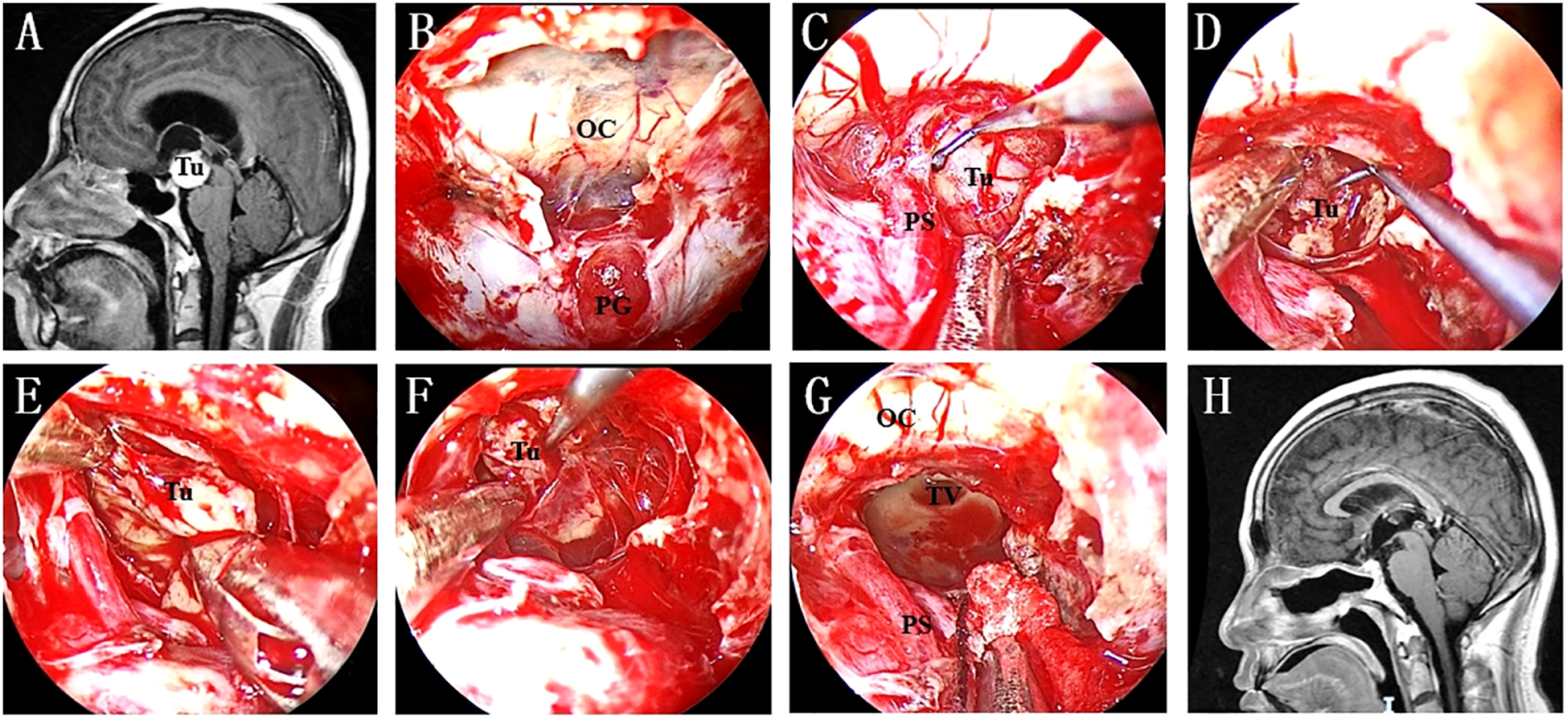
(A) Preoperative MR image showing a lesion in the retroinfundibular area. (B) A “Y”-shaped dural incision was made to expose the PG and optic chiasma. (C) The lesion was exposed after intradural PGT and clival dura opening. (D) Intratumoral debulking was performed as the first step to ensure extensive tumour resection. (E) The medial interface was identified after enough tumour debulking. (F) Inferior extracapsular dissection was continued to achieve radical tumour resection. (G) The TV was opened after the tumour was completely resected. (H) No residual tumour or recurrence was found on postoperative MRI. Tu, tumour; OC, optic chiasma; PG, pituitary gland; PS, pituitary stalk; TV, third ventricle.
